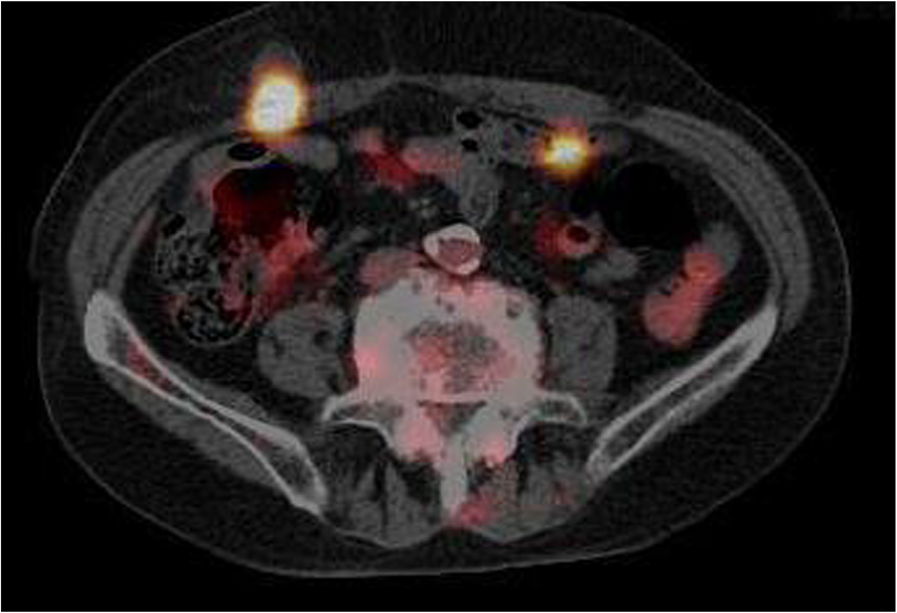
Pre-operative PET-CT showed high accumulation of FDG (SUV_max_ 14.6) at the ventral side of the right abdominal wall
